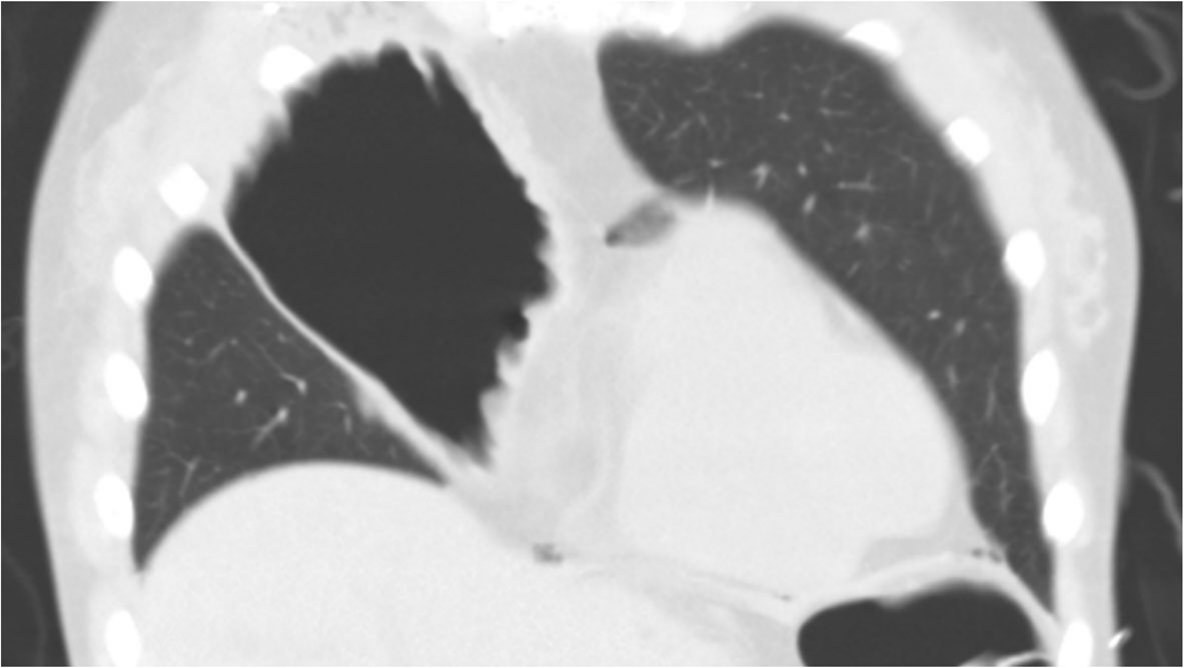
Chest computed tomographic image showing coronal section of the distended, reconstructed whole stomach compressing the proximal lung parenchyma
